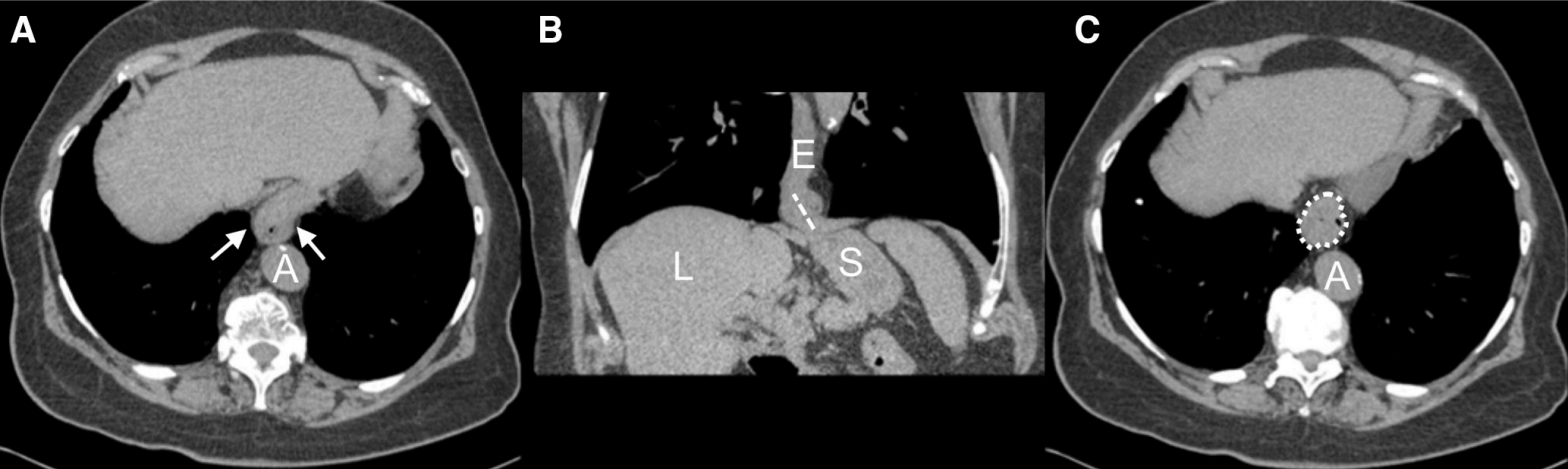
Non-contrast chest CT of a woman aged 84 years. (A) Axial image showing type I hiatal hernia (white arrows). (B) Coronal oblique reformation shows gastric folds extending 2.9 cm (dashed line) above the diaphragm. (C) Axial image shows maximum hiatal hernia cross-sectional area measurement (dotted line, 7.6 cm^2^). A, aorta; E, oesophagus; L, liver; S, stomach.
